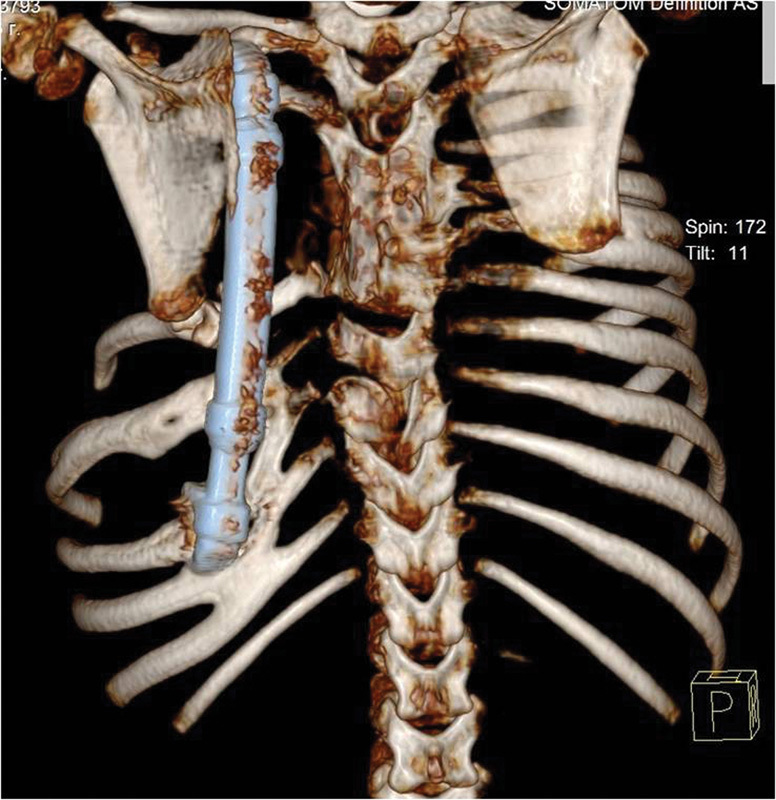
3D CT reconstruction image of the chest with VEPTR device in place, 1
